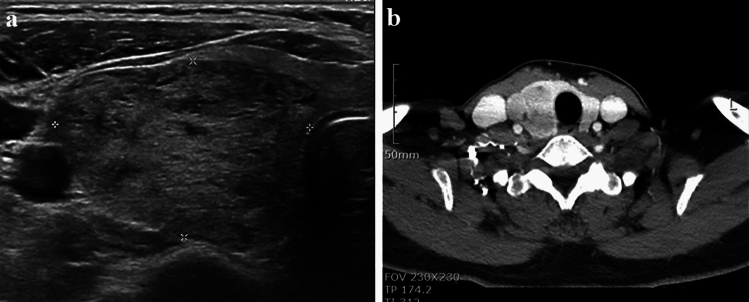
Preoperative ultrasonographic and neck computed tomographic image for an 56-year-old female patient with 4.2 cm goiter. (a) Ultrasonographic finding of thyroid goiter. There was suspicious 3.3 × 4.2 cm hypoechoic lesion in lower pole of right thyroid and no significant cervical lymph node enlargement. (b) CT finding of a 4.0 cm enhancing goiter in right thyroid gland.
